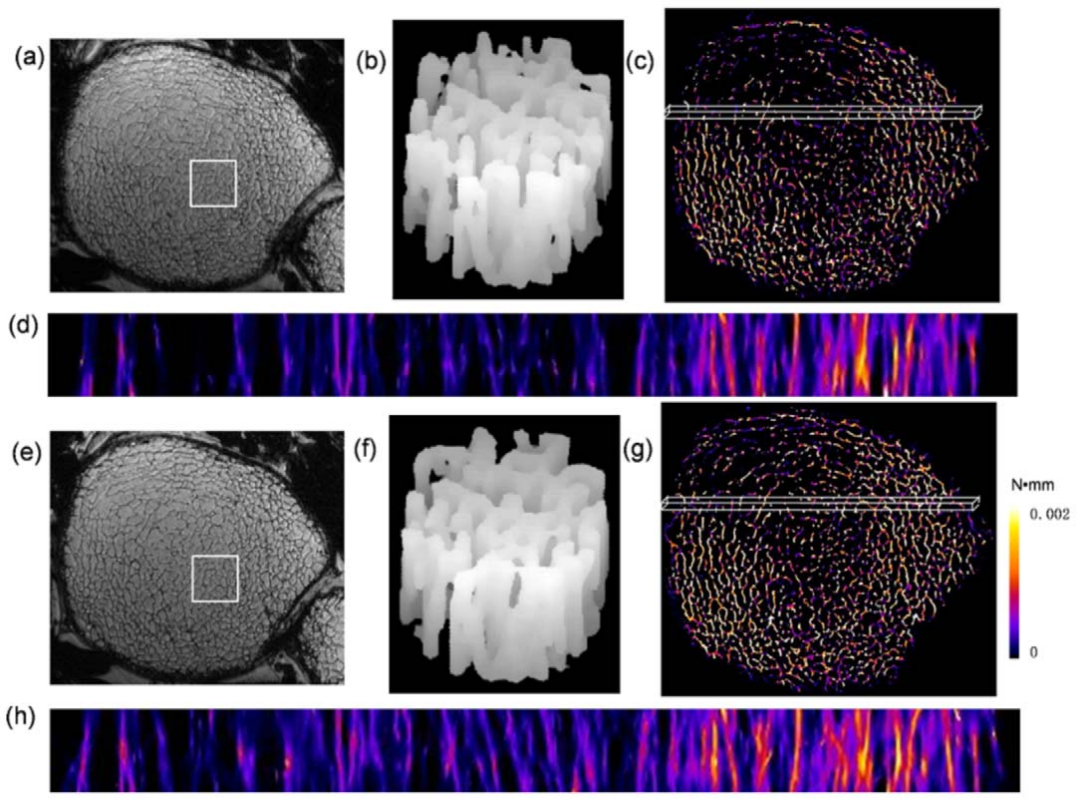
Structural MR images and resulting strain energy maps from two 3D data sets taken at 7T field strength at the left distal femur in a patient treated with zoledronic acid (Reclast™) for a period of 12 months. a, e) cross-sectional images from stack of contiguous slices at baseline and after 12 months of treatment; b, f) volume-renderings of square-shaped sub-regions (7.8×7.8×3.7 mm^3^) indicated in images a and e, highlighting structural similarity at the two time-points; d, h) longitudinal projections of strain-energy data displayed for a thin slab (1.1×37.7×3.7 mm^3^) in the anterior region indicated in c and g.
